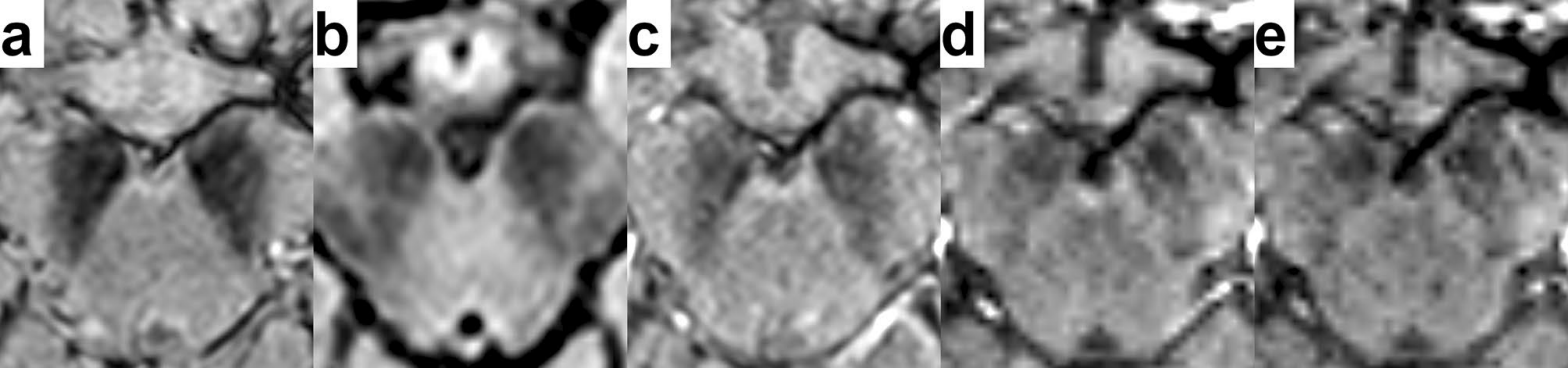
True-positive interpretation of the images of a 62-year-old woman with PD (Hoehn-Yahr stage: 2, MDS-UPDRS Part II: 6 points, MDS-UPDRS Part III: 24 points). Nigral hyperintensity was bilaterally rated as “not visible” on EPISEG (a), FLAIR (b), MEDIC (c), MAG (d), and SWI (e) images as well. Images are shown in radiological convention (right = subject’s left) at a level below the red nucleus.
